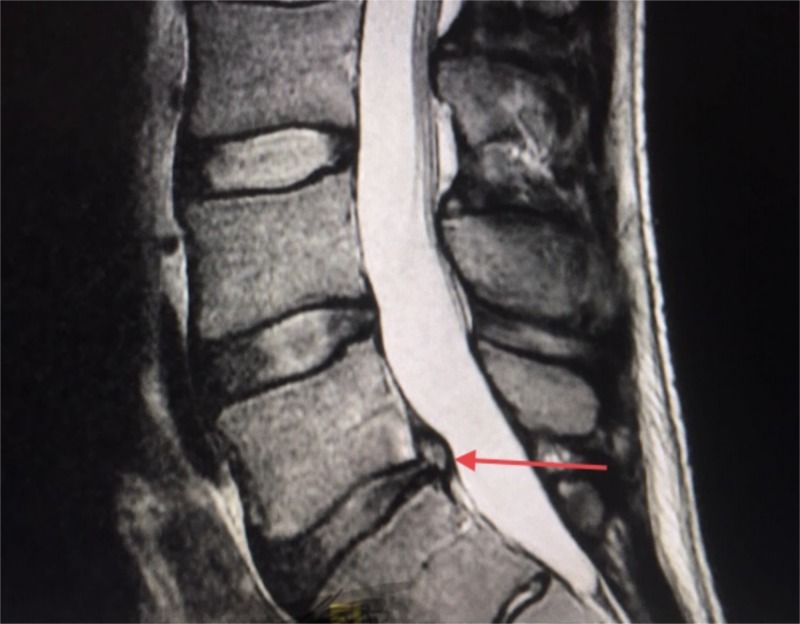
Magnetic resonance imaging of the lumbar spine Disc posterior herniation (red arrow)
